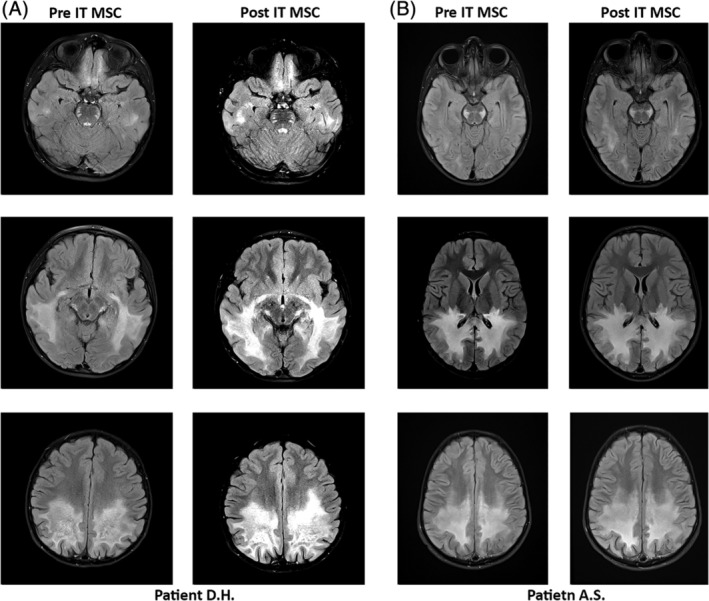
Magnetic resonance images (MRIs) before and after mesenchymal stem cells (MSCs). A, Patient D.H. MRI was 2 months following the third intrathecal (IT) MSC dose. B, Patient A.S. MRI was 1 month after the dose of IT MSCs
